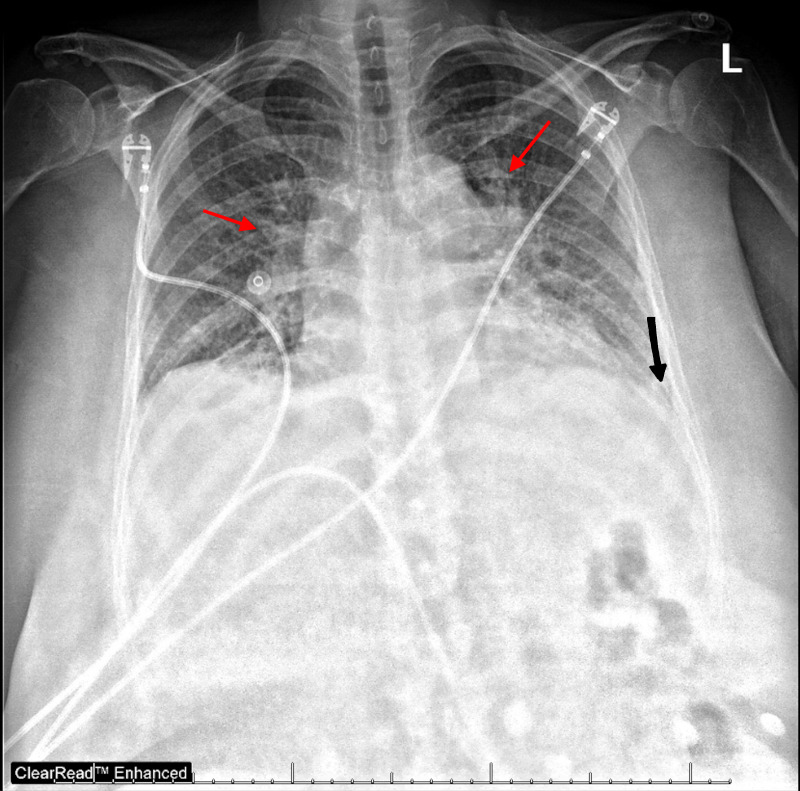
Chest radiograph showing pulmonary edema (red arrows) and small pleural
